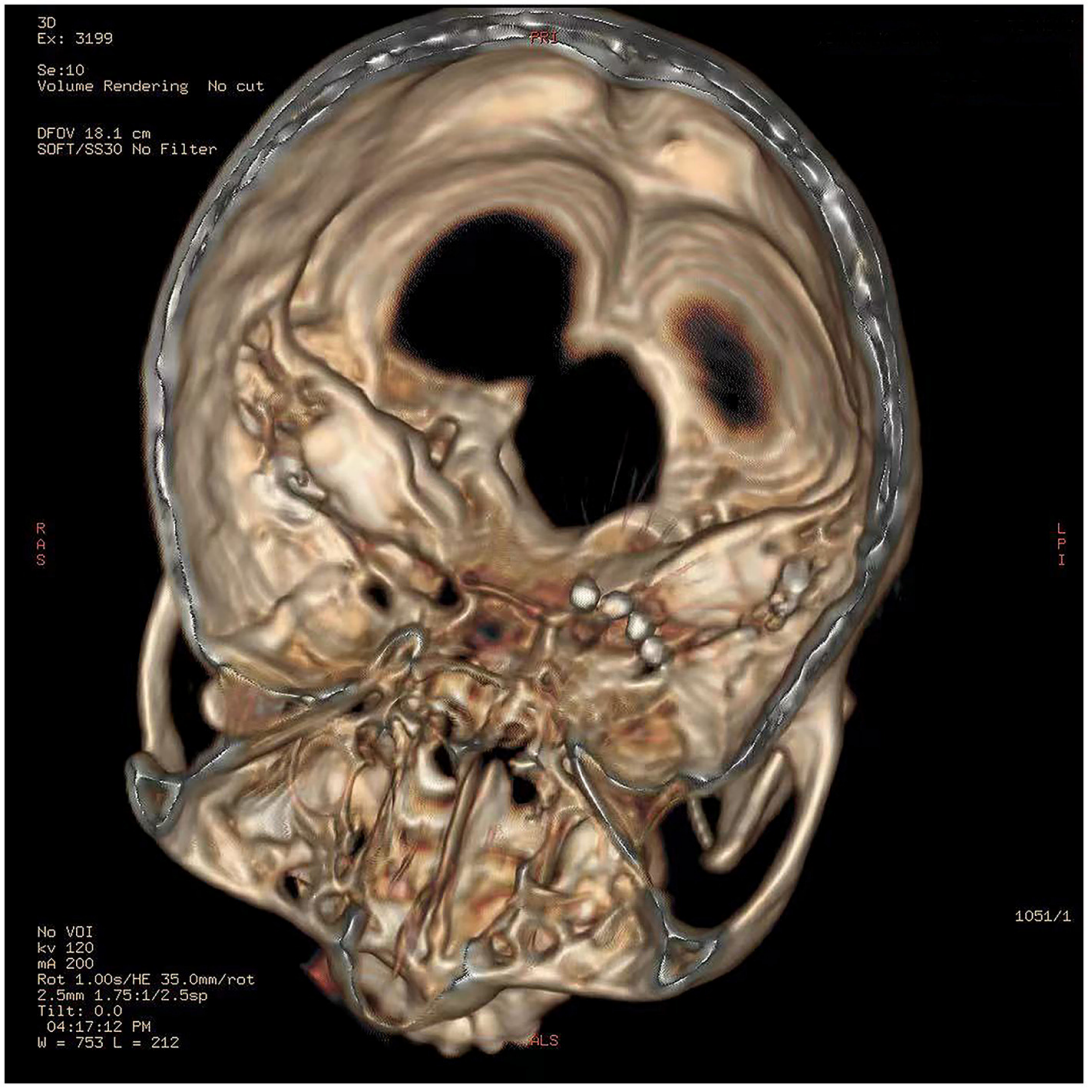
The electrode remained in the correct position under CT reconstruction.
